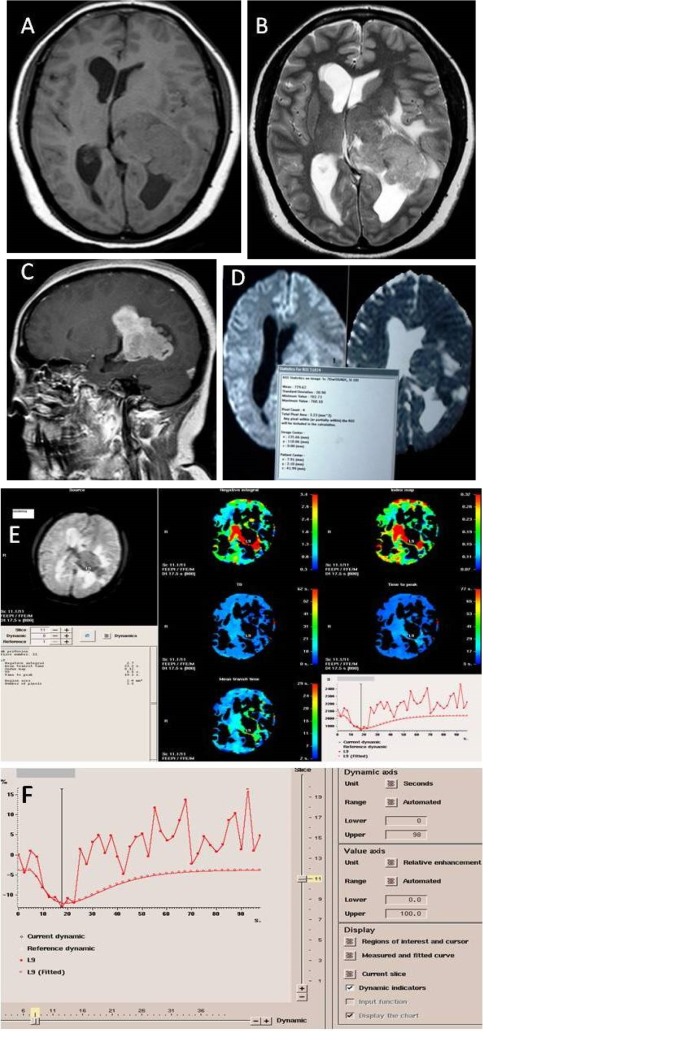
A 40-year-old female with left lateral ventricle meningioma (malignant) A. Axial T1W image showing hypointense lesion arising from left lateral ventricle B. T2W image showing iso- to hyper-intense lesion with perilesional edema causing expansion of left lateral ventricle C. On contrast enhanced saggital T1W image the lesion shows homogenous intense enhancement D. Axial DWI and corrosponding ADC map showing diffusion restriction in its solid part with minimum ADC value being 0.729×10^−3^ mm^2^/_s_ E. T2W* first pass perfusion images showing colour coded rCBV maps along with various parameters with rCBV (negative integral) in peitumoral edema being 2.7 mL/100 g F. Time signal intensity curve in peritumoral edema showing maximum signal intensity drop of 15%
